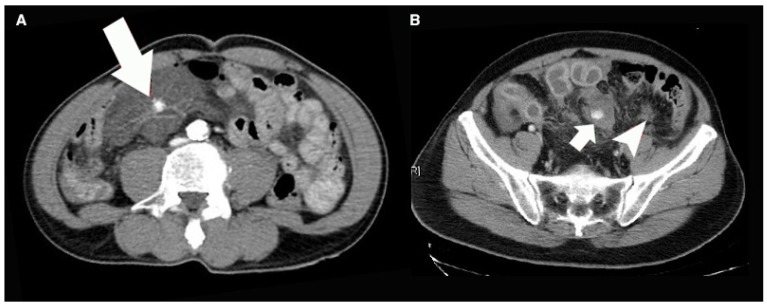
(A) The axial contrast-enhanced abdominal computed tomography showed the mesenteric injury with focal hematoma and mesenteric pseudoaneurysm (arrow). (B) The axial contrast-enhanced computed tomography revealed multiple mesenteric lacerations present as mesenteric extravasation (arrow) and mesenteric infiltration (arrowhead).
